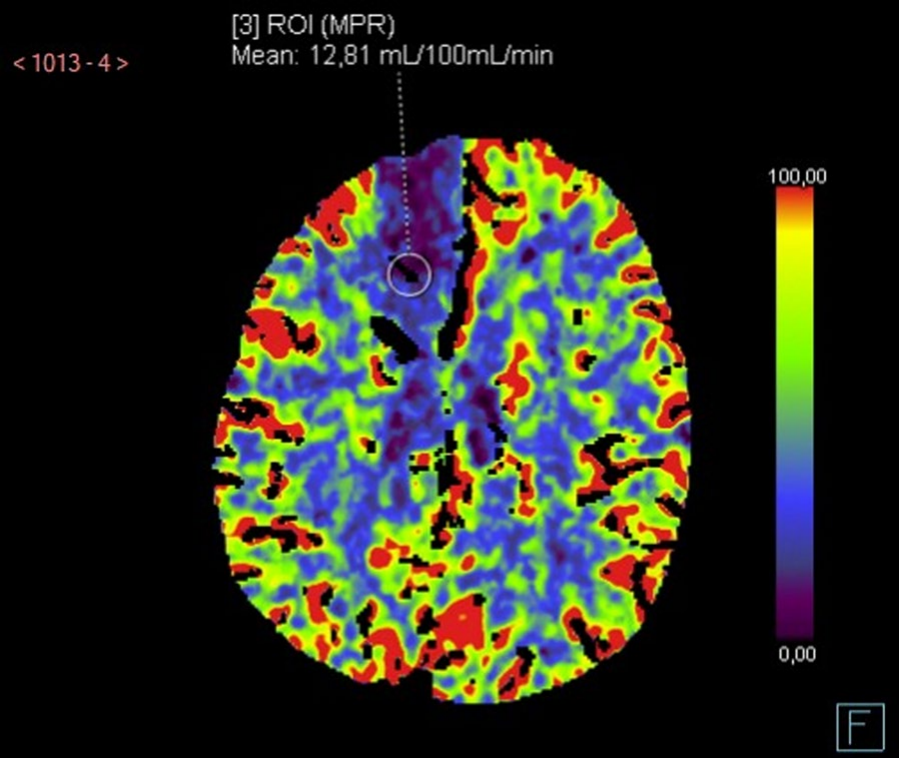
Patient with severe subarachnoid hemorrhage, who underwent a cerebral CT-perfusion (CTP) on day 2. White circle indicated the region of interest (ROI) for CTP analysis of regional cerebral blood flow (rCBF); rCBF was estimated at 12.8 mL/100 g × min, while intracranial pressure and cerebral perfusion pressure were 16 mmHg and 73 mmHg, respectively, and baseline PbtO_2_ was 22 mmHg (for a PaO_2_ of 119 mmHg). Measured OxR was 0.14
